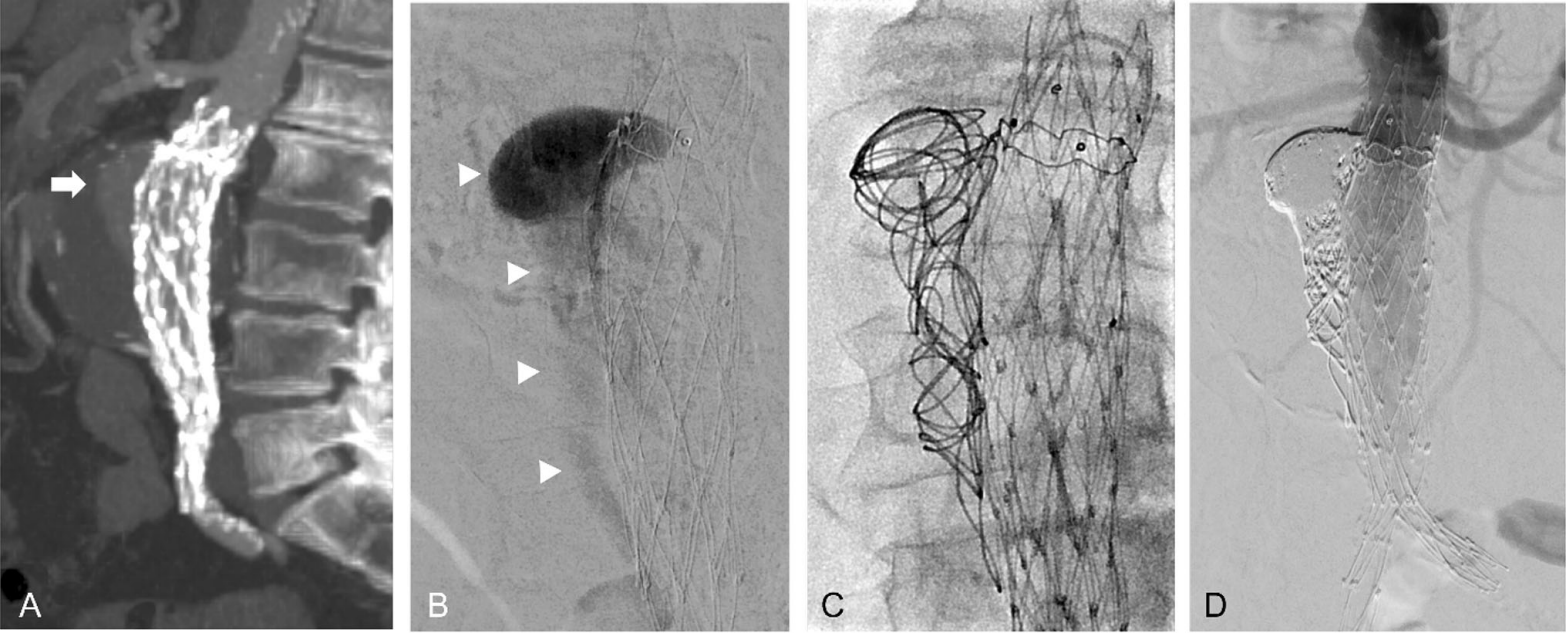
Type IA EL embolisation. (A) Sagittal CT image shows proximal type I EL post-EVAR (arrow). Unsuitable for treatment with an aortic cuff due to heavily diseased iliac arteries. (B) Entry channel between aortic wall and stent graft was engaged with a reverse-curve catheter. The subsequent angiogram outlines a large EL cavity (arrows). (C) Embolisation of EL cavity with coils via a microcatheter. (D) Completion angiogram shows successful embolisation
